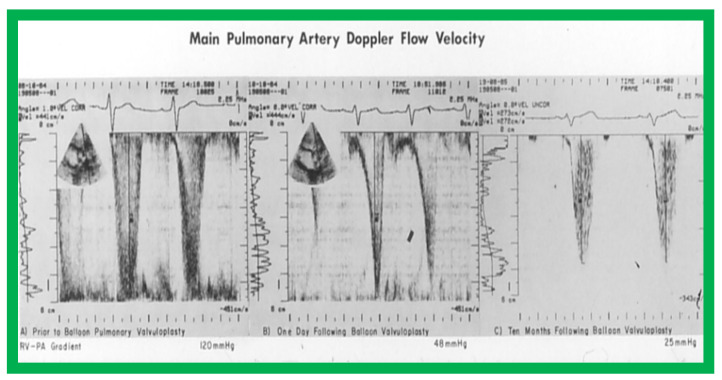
The main pulmonary artery Doppler flow velocities prior to (A), and one day (B) and ten months (C) following balloon pulmonary valvuloplasty are shown. Note that there is a significant fall in the peak flow velocity immediately after valvuloplasty, but a moderate (48 mmHg) gradient that has a characteristic triangular pattern, highly suggestive of infundibular obstruction (corresponding to Figure 2A) persisted. At 10-month follow-up, the flow velocity has markedly diminished, indicating the resolution of the infundibular obstruction (corresponding to Figure 2B). The residual calculated gradients are shown at the bottom of each panel. Reproduced from Reference [4].
